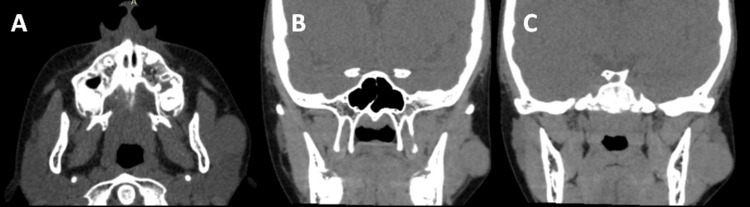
CT scan showing an isodense image corresponding to the lesion. Isodense image overlying the left parotid gland and masseter muscle and underlying cutaneous tissue, of 2.56 cm in larger diameter, with well-defined margins throughout its extent, corresponding to a tumor lesion. Axial (a) and coronal view (b and c).
